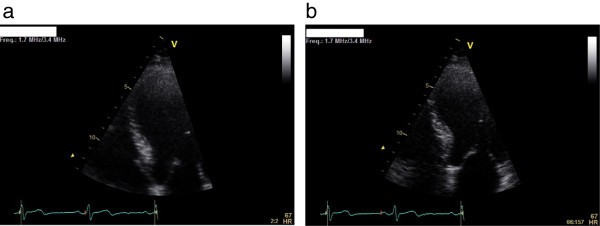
Transthoracic echocardiography. Apical two-chamber view in (a) systole and (b) diastole. No evidence of mass is seen in this view from 12 months previously. V is a marker which shows where the transducer is.
